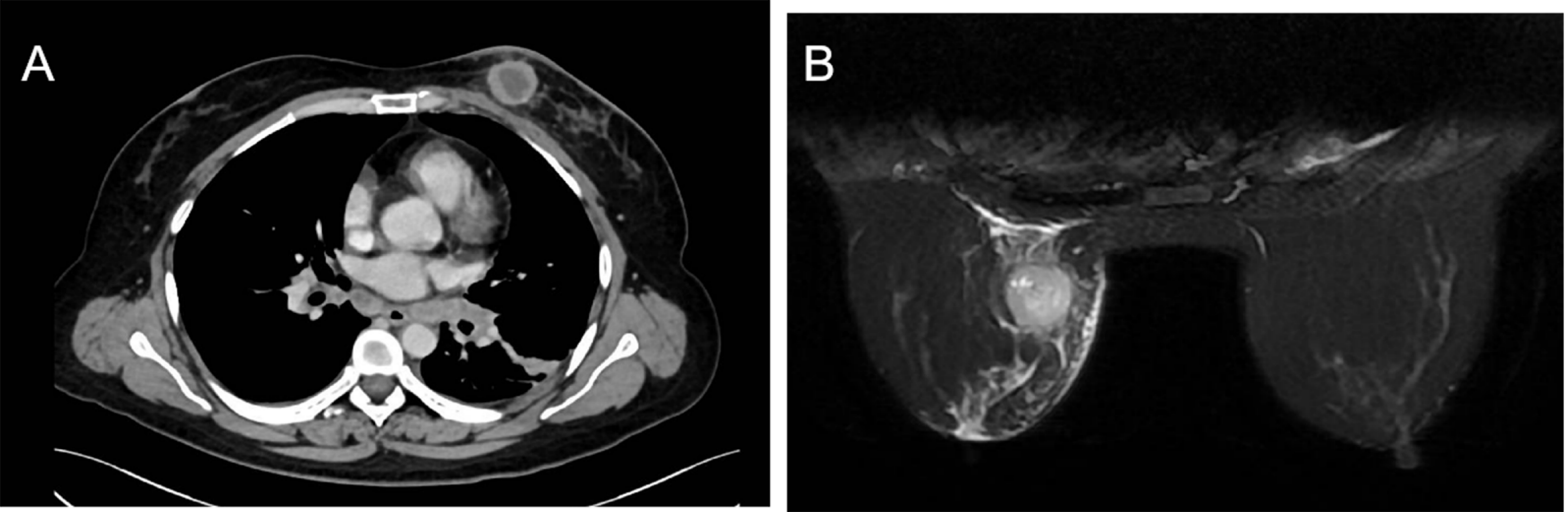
Chest CT. Chest CT before treatment (A). Chest MRI before treatment (B).
